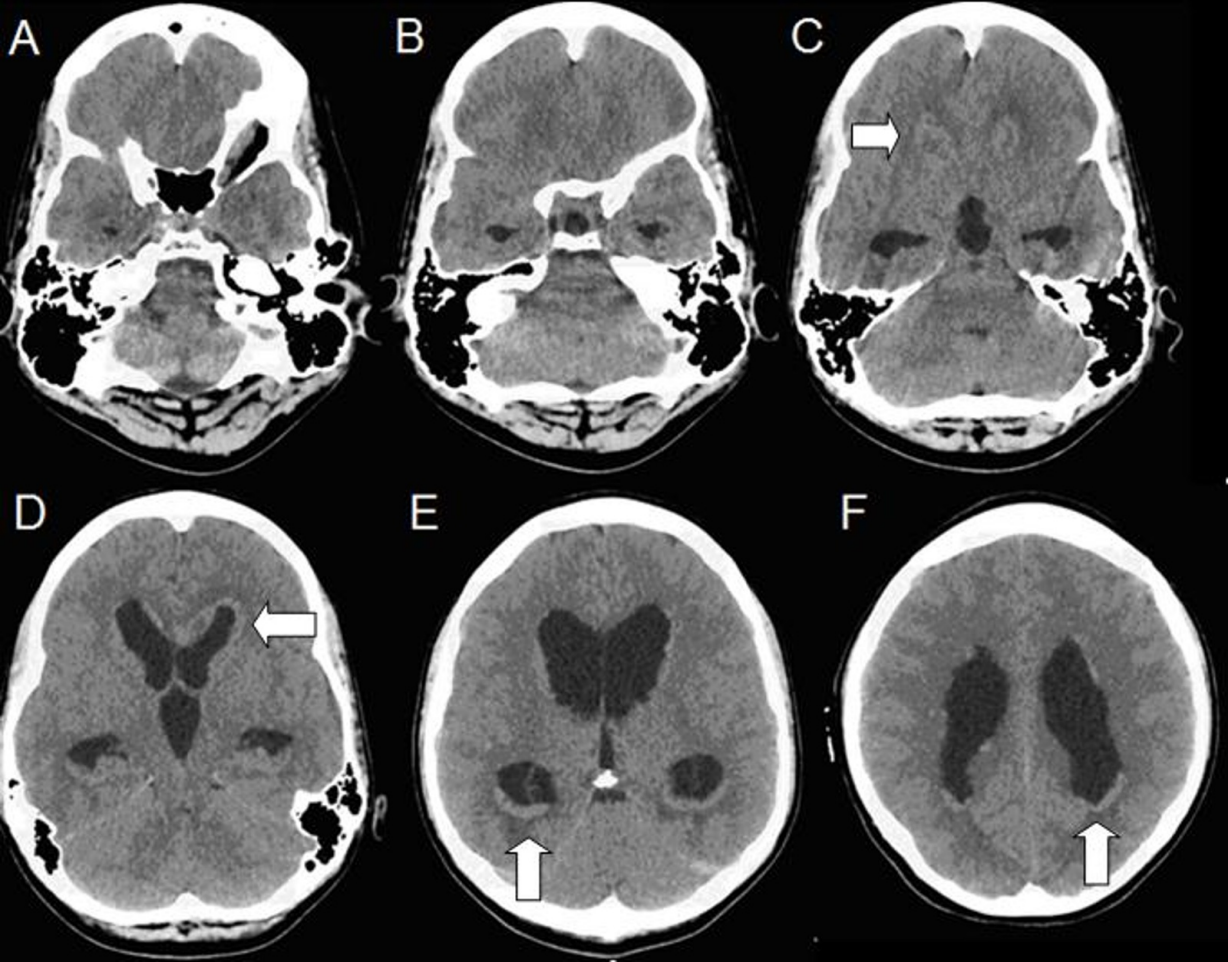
Non-contrast-enhanced CT head at initial presentation. There is transependymal fluid shift with enlargement of the lateral and third ventricles with nodular densities prominent in the occipital and anterior horns bilaterally.
